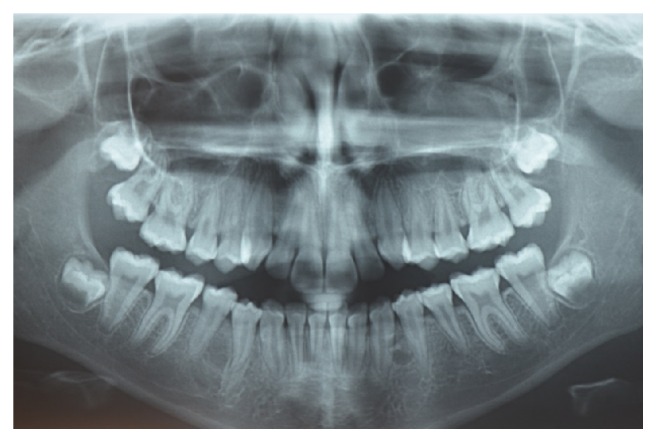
Panoramic radiograph of the patient aged 11 years and 3 months showing the early formation of a supplemental supernumerary tooth in the left mandibular premolar region.
